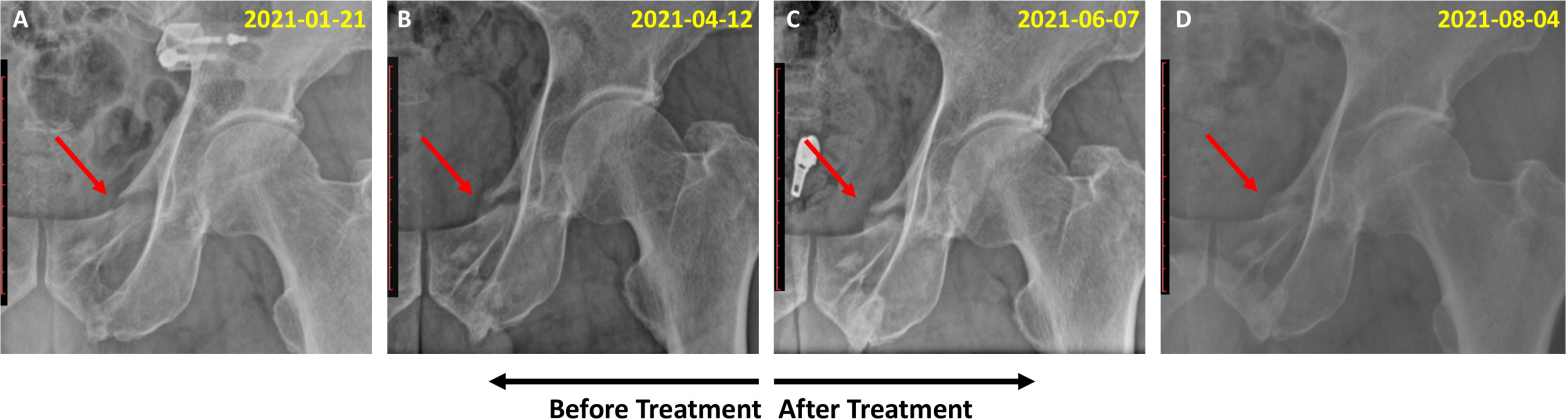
Radiographic images of the pelvis of Case 1, a 65-year-old man with a pubic ramus fracture. (A) Fracture condition of the pubic ramus at 3 months after the traffic accident (January 21, 2021); (B) Callus formation status 6 months after the fracture (April 12, 2021); (C) Soft callus formation 2 months after JGT administration (June 7, 2021); (D) Hard callus formation (August 4, 2021).
